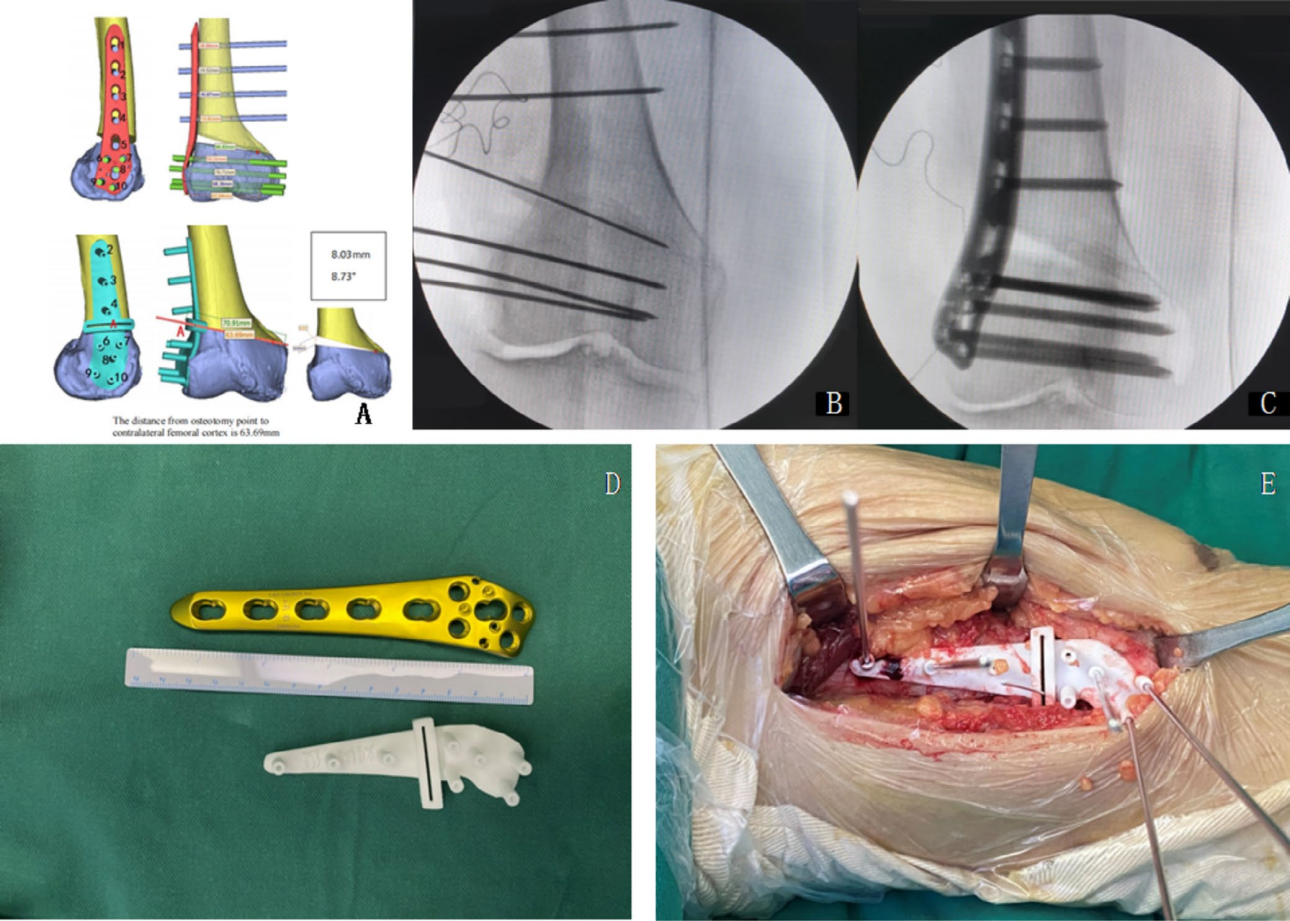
(A) 3D osteotomy planning based on preoperative CT scan. (B) Intraoperative fluoroscopic images. Adjustment to the optimal osteotomy position according to intraoperative fluoroscopic images. (C) Intraoperative fluoroscopic images. The bone is cut, and the gap is opened, plate screws are placed, and intraoperative fluoroscopy is performed to ensure surgical precision. (D) Titanium plate and 3D patient‐specific printed cutting guide used in the surgery procedure. (E) Intraoperative use of 3D patient‐specific printed cutting guide to determine the direction of osteotomy.
